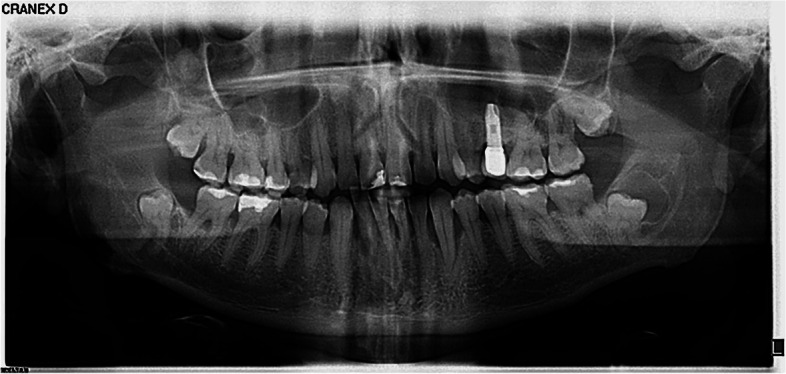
Panoramic radiograph number 8 used in the survey for evaluation of MTM 48
